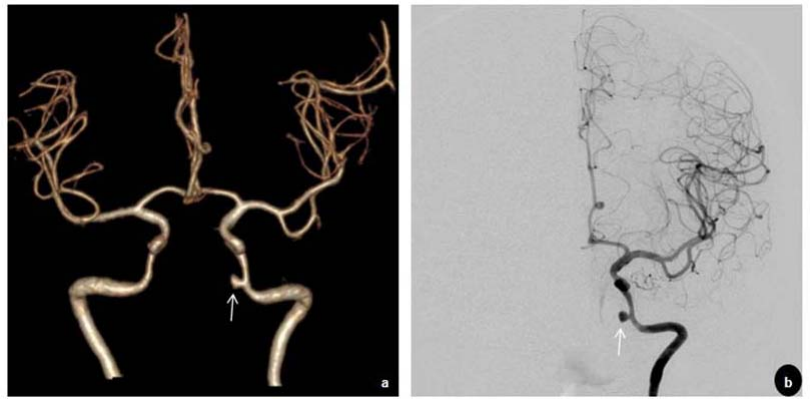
A capsule-like protrusion (arrow) at the internal carotid artery is identified on the computed tomography angiography (a) and digital subtraction angiography images (b).
